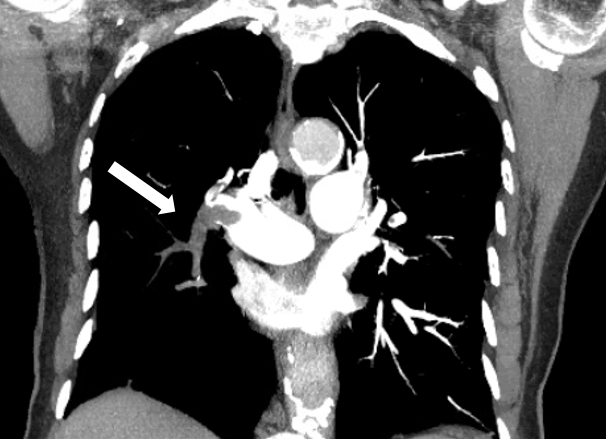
Coronal view of the computed tomography pulmonary angiogram. Image is notable for pulmonary embolus within right pulmonary artery trunk with almost complete occlusion of segmental branches (arrow).
